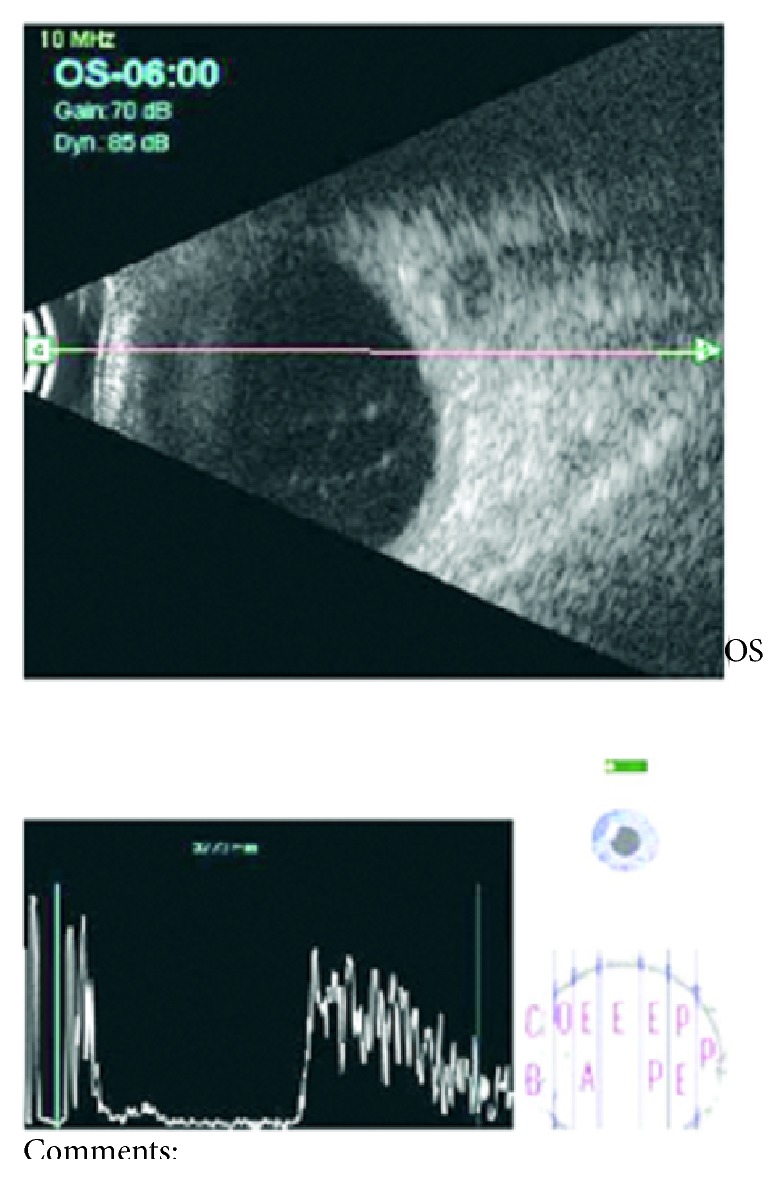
Ultrasound showing fine vitreous opacities with flat retina.
